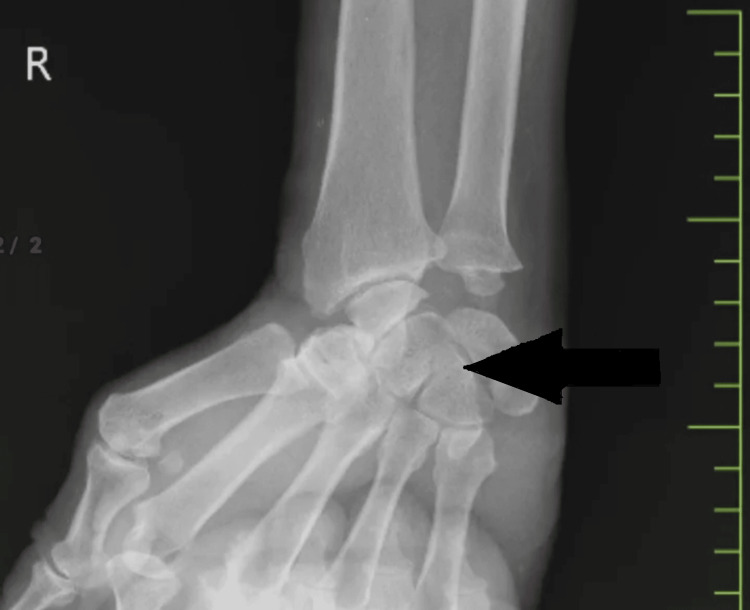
Pre-operative X-ray X-ray showing the right wrist joint. X-ray was taken in antero-posterior view before the operation
